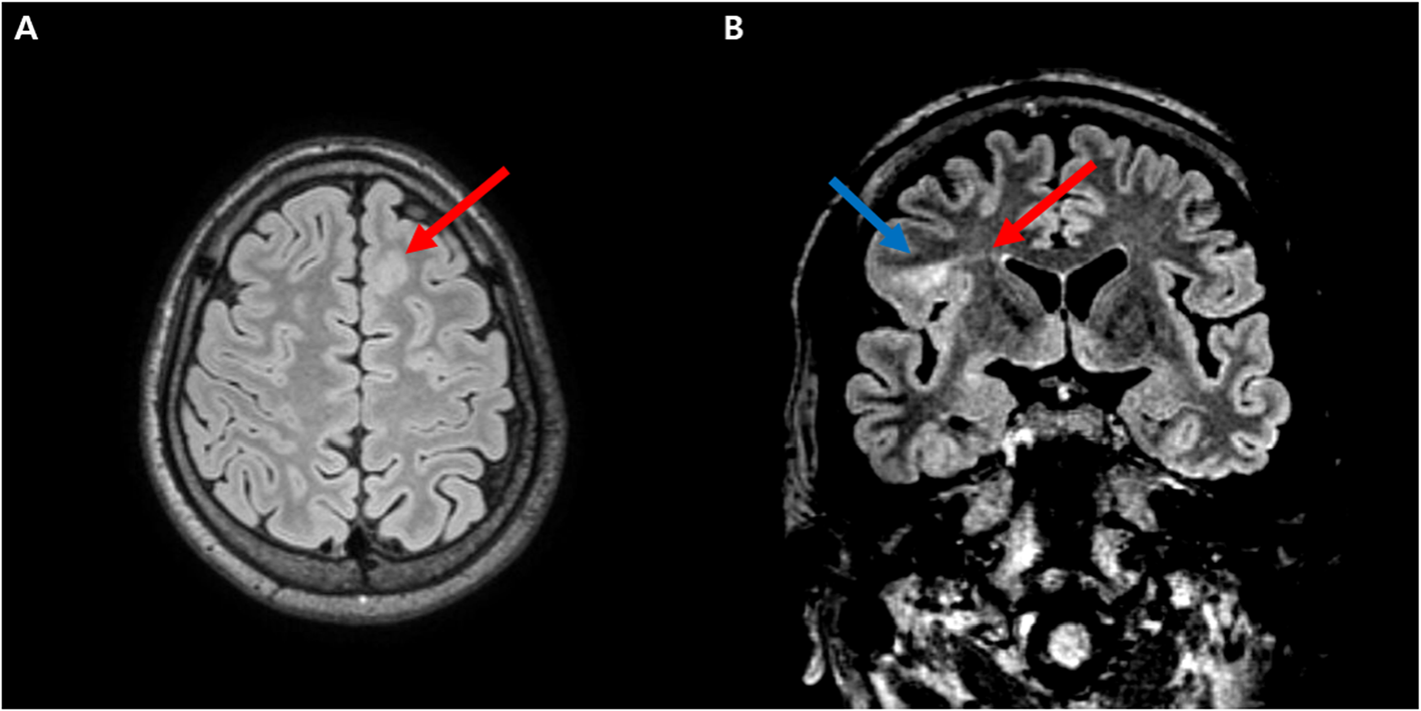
An example of typical brain MRI findings compatible with a focal cortical dysplasia. Fluid-attenuated inversion recovery (FLAIR) MRI of a patient showing focal cortical thickening with blurring of gray-white matter differentiation in the left frontal lobe (red arrow) (A). FLAIR MRI of another patient reveals focal increased signal intensity (blue arrow) with transmantle sign (red arrow) in the right frontal lobe (B)
